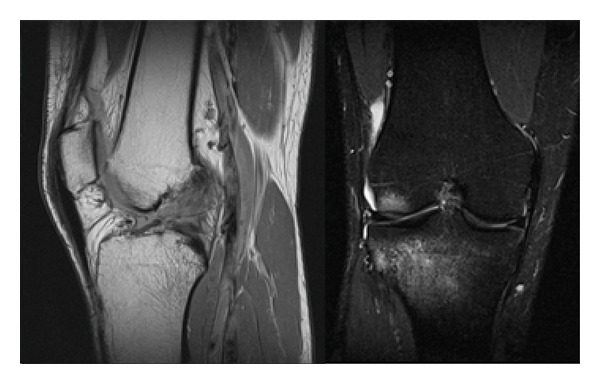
Sagittal T1-weighted MRI shows ACL lesion. Coronal T2 weighted shows hyperintense imaging in the external tibial plateau and lateral femoral condyle.
